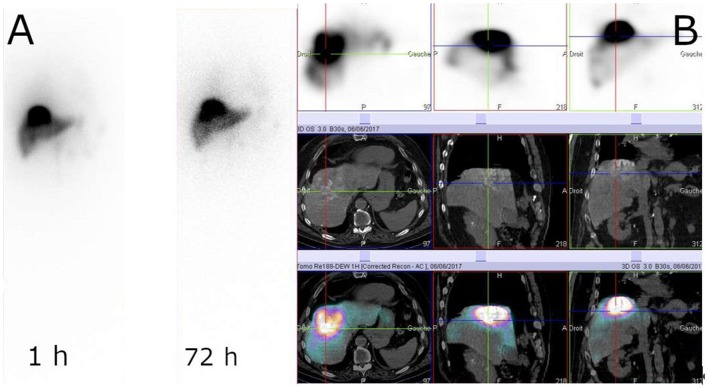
Example of ^188^Re-SSS biodistribution profile. Whole-body scintigraphy at 1 and 72 h (A) and SPECT/CT at 1 h (B) (Courtesy of Prof. Etienne Garin, Rennes, France).
